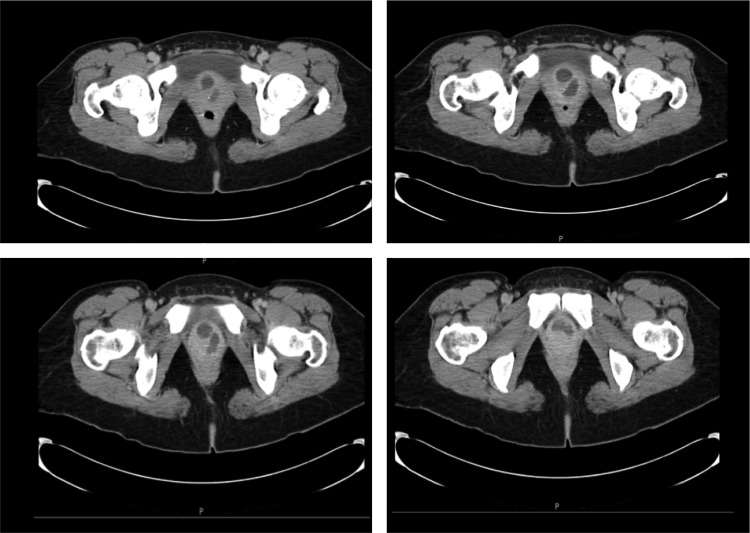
CT image of the large, proximal, and anterior urethral diverticulum that wraps around the urethra and bladder neck in a complex horseshoe configuration.
